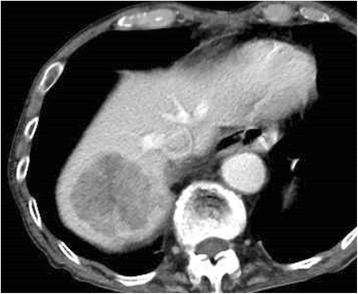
Abdominal computed tomography (CT) findings 2 months after surgery. A liver tumor was identified with enhanced delivery at the edges implying high external density and low intracellular density
